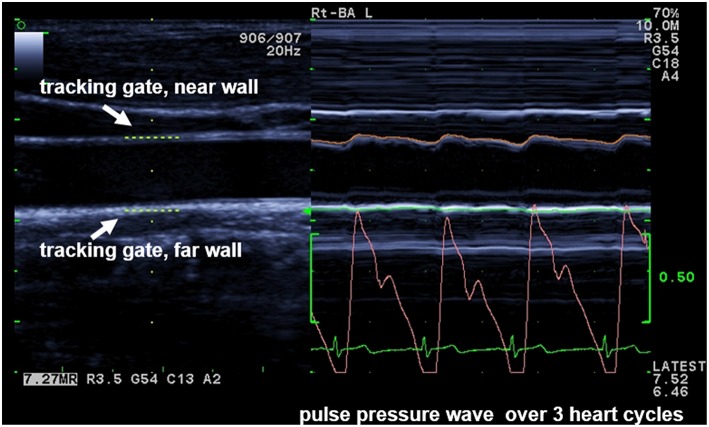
Ultrasound echo tracking (ET) PWV measurement at the common carotid artery.
